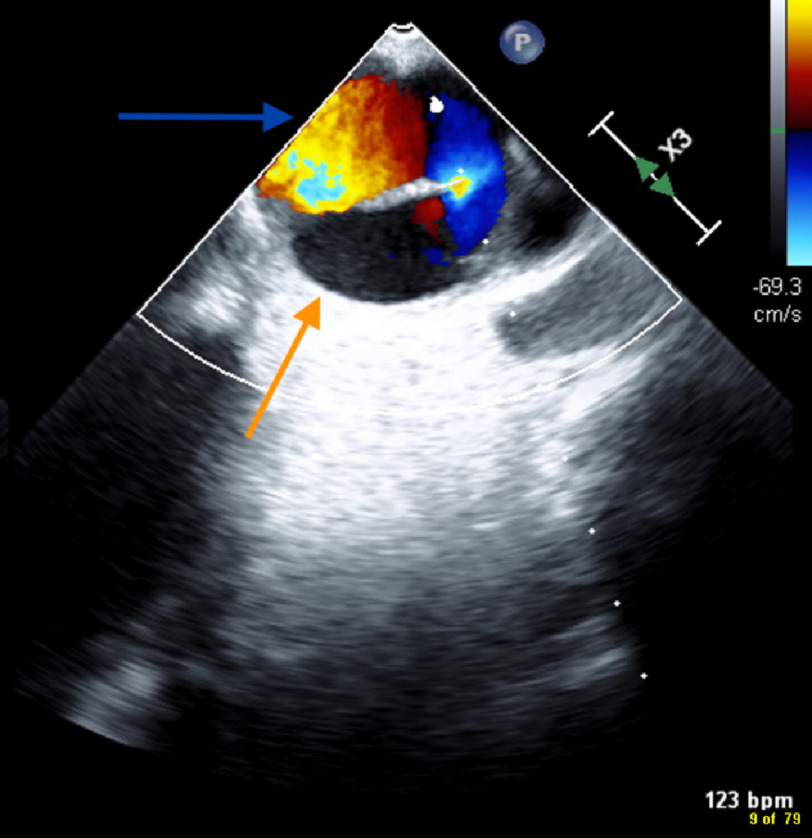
Chronic Stanford Type A aortic dissection on transesophageal echocardiogram with color flow doppler showing true lumen (blue arrow) and false lumen (orange arrow).
